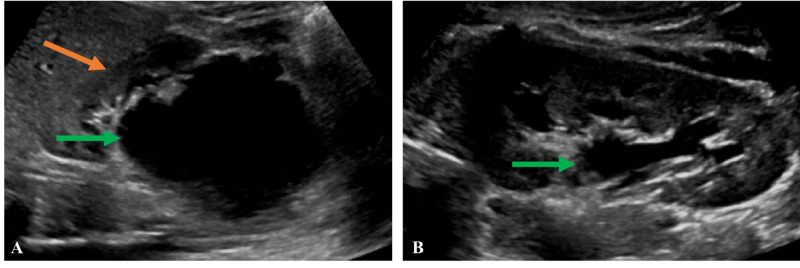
Follow-up renal ultrasound (A) Right kidney with severe hydronephrosis (green arrow) with associated parenchymal thinning and echogenic renal parenchyma (orange arrow). (B) Left kidney with stable moderate hydronephrosis (green arrow).
